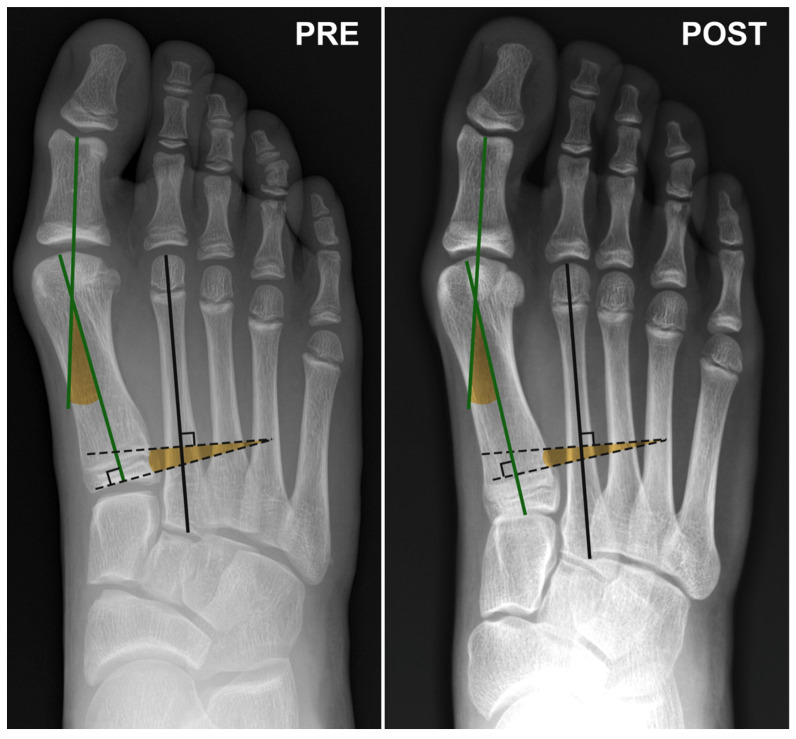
Dorsoplantar X-ray view of the foot of an 11-year-old female patient at the time of surgery and at the 6-month postoperative follow-up. Angle subtended by the green lines: Hallux Valgus Angle (HVA); Angle subtended by the black dashed lines: InterMetatarsal Angle (IMA).
